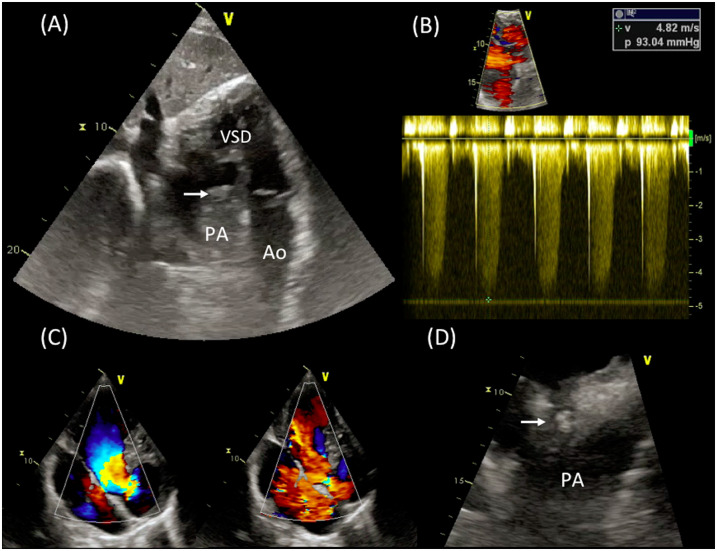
Pulmonary valve endocarditis in a patient with complex cardiac diagnosis including congenitally corrected transposition of the great arteries and pulmonary stenosis treated with balloon pulmonary valve dilatation (Case 2). (*A*) Subcostal view demonstrating parallel course of the great arteries with stenotic pulmonary valve overriding an unrestricted ventricular septal defect. An echogenic mobile vegetation attached to the ventricular side of the pulmonary valve is also seen (arrow). (*B*) Continuous wave Doppler signal showing pulmonary valve transvalvular gradient. (*C*) Bidirectional flow across the subpulmonary ventricular septal defect. (*D*) Zoomed view on the pulmonary valve mass from a modified apical view. Ao, aorta; Arrow, vegetation; PA, pulmonary artery; PV, pulmonary valve; VSD, ventricular septal defect.
